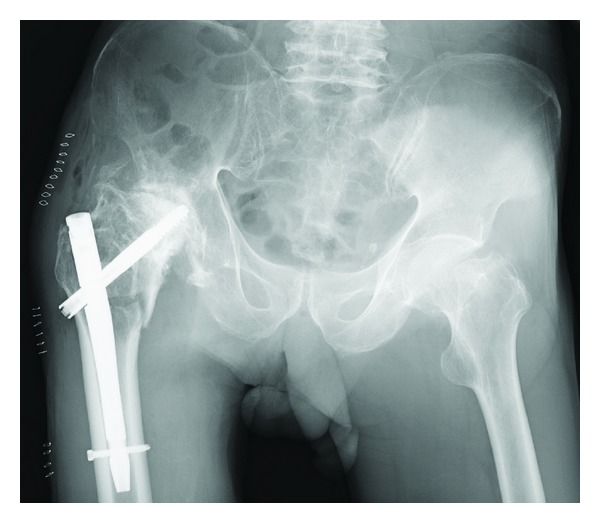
Anteroposterior radiograph captured after the operation showing the intertrochanteric fracture stabilized with a gamma nail.
